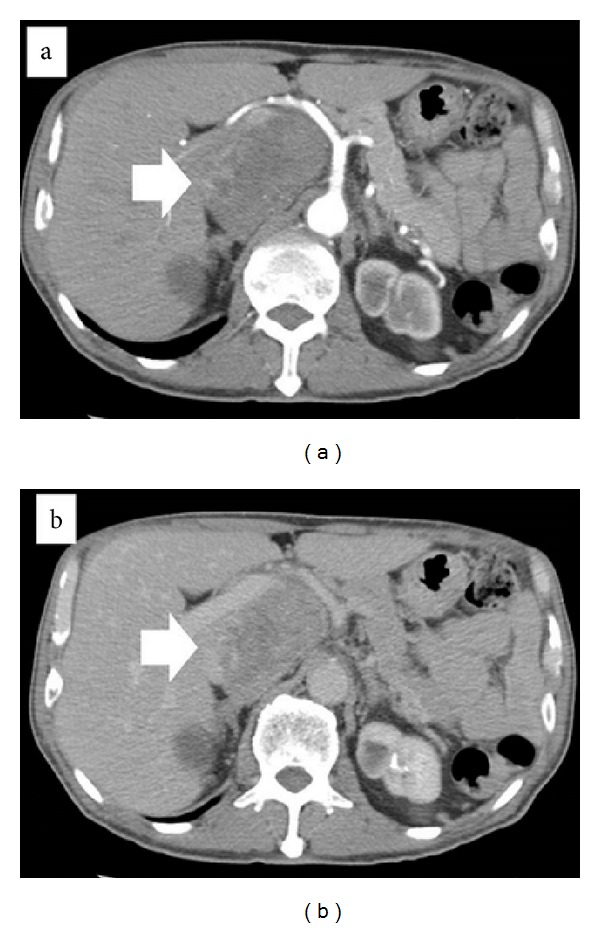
Enhanced computed tomography (CT) of the abdomen revealed a slightly enhanced heterogeneous tumor, 18 mm in diameter, between the Spiegel lobe of the liver and the IVC in early-phase images ((a) arrow), with no enhancement and washout in late-phase images ((b) arrow).
